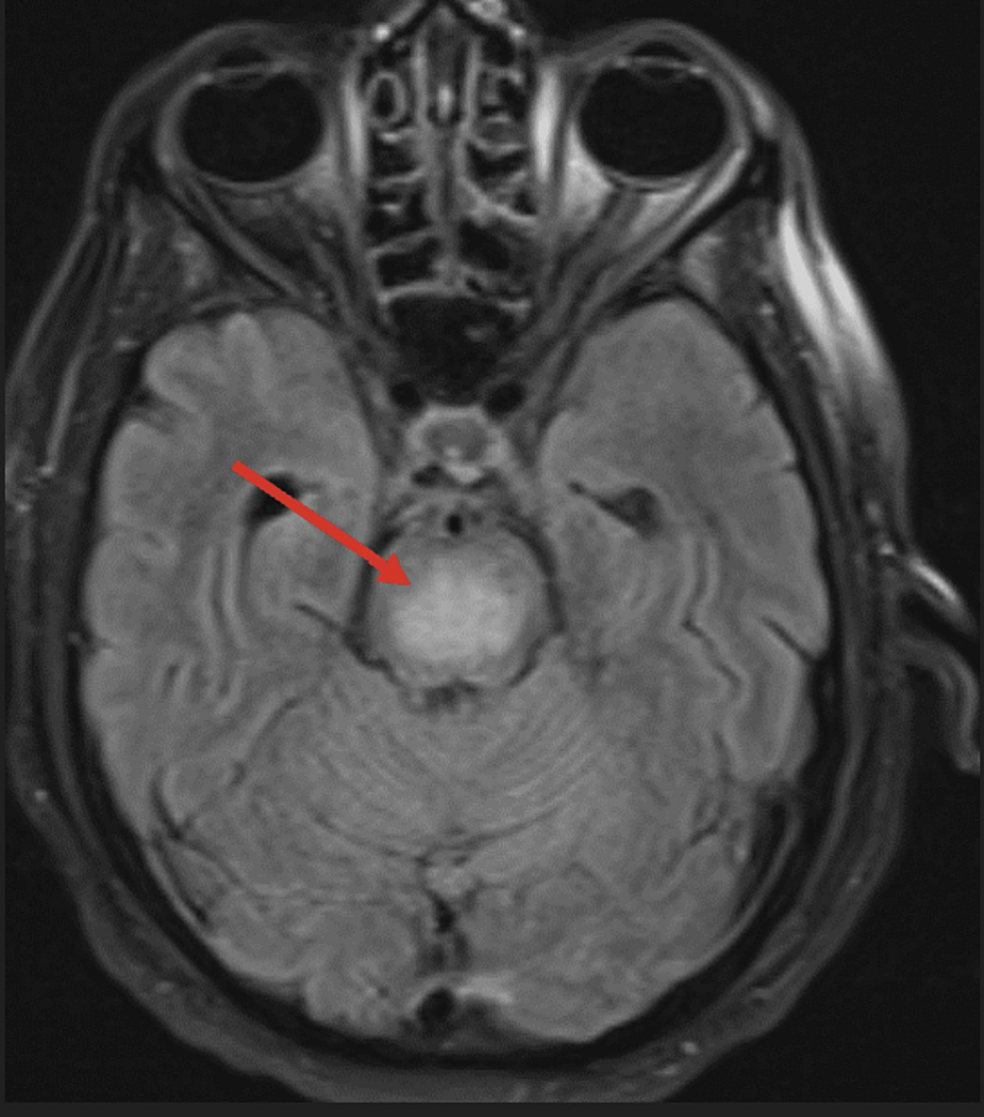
Brain MRI FLAIR sequence showing CPM in a patient with GAD antibody (red arrow). CPM: central pontine myelinolysis; FLAIR: fluid-attenuated inversion recovery; GAD: glutamic acid decarboxylase.
